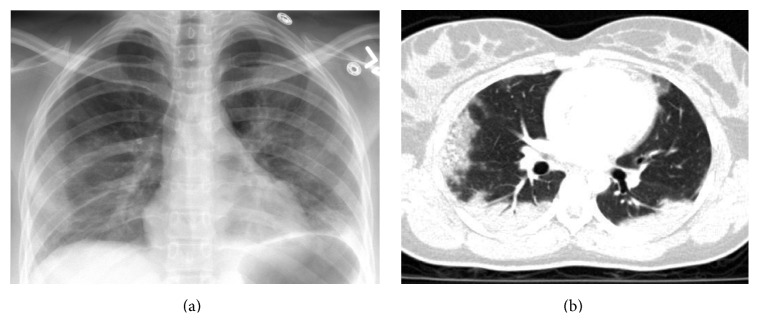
(a) Portable chest X-ray showing bilateral lung opacities with relative sparing of the right upper lobe and left pleural effusions. (b) CT scan of chest showing multifocal and bilateral, predominantly peripheral airspace opacities.
